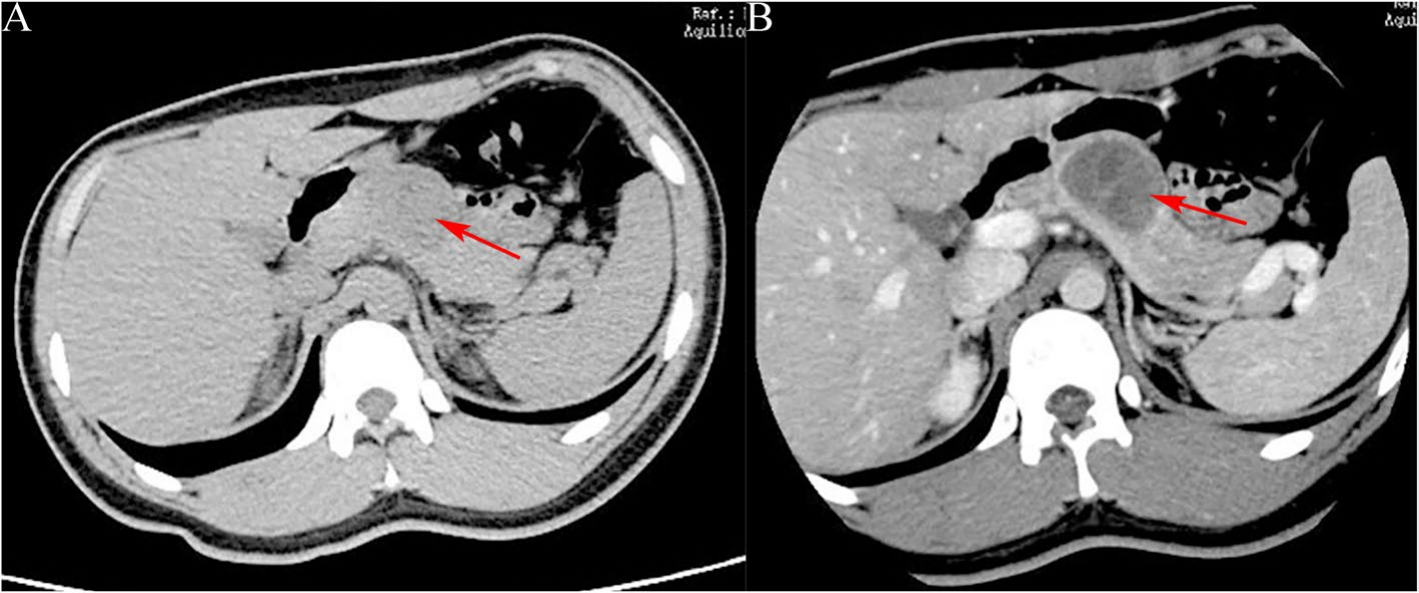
A 17-year-old male with solid pseudopapillary tumor. a Plain CT scanning shows a quasi-circular slightly lower density shadow of the pancreatic body (long arrow); b CT target scanning shows that the tumor capsule is complete and enhanced solid components (long arrow) are seen on the margin during the pancreatic parenchymal phase
